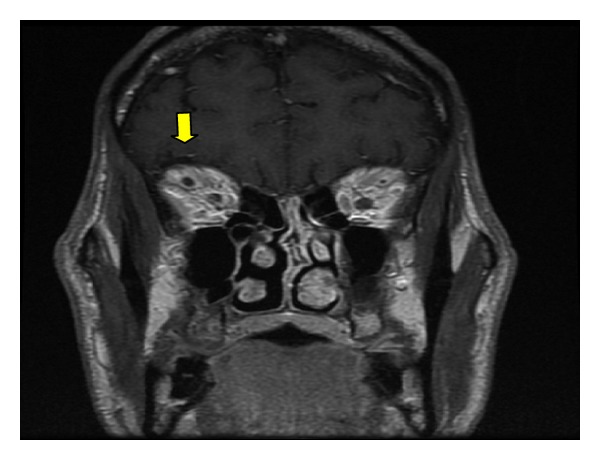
Head MRI with enlarged right superior ophthalmic vein.
